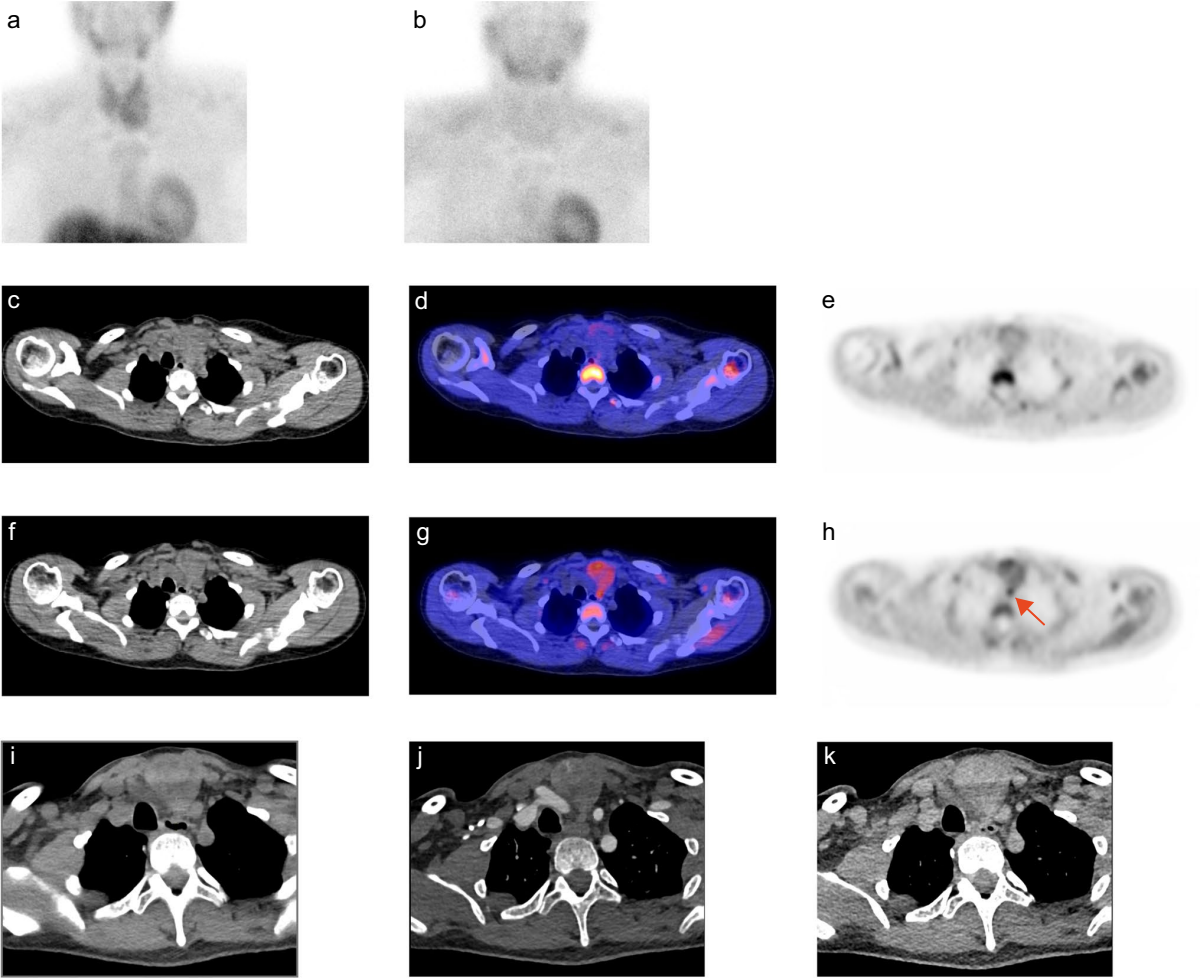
Preoperative parathyroid imaging of a representative patient. Planar anterior image of the neck with [^99m^Tc]Tc-MIBI (a early, b late) does not localize the parathyroid adenoma. The [^11^C]methionine PET/CT (c ldCT image, d fused PET/CT image, and e PET only image) also does not clearly locate a parathyroid adenoma (negative at both the blinded and non-blinded interpretation). On the [^11^C]choline PET/CT (f ldCT image, g fused PET/CT image, and h PET only image), a lesion suspicious for a parathyroid adenoma was shown caudally to the left thyroid lobe (red arrow in h). During parathyroidectomy, a parathyroid adenoma (0.9 cm and 0.18 g at pathology) was removed from this exact location. The 4D-CT scan (i nonenhanced phase, j arterial phase, k venous phase) did not evidently show the classic characteristic contrast enhancement pattern of parathyroid adenoma and was therefore declared as negative based on the blinded and non-blinded interpretation
